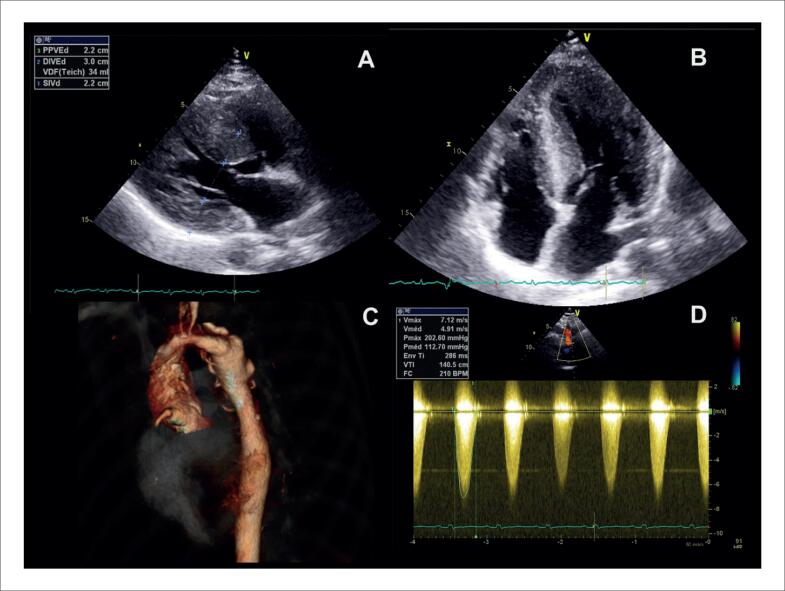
Imagens ecocardiográficas (A, B e D) e de angiotomografia de aorta torácica (C) de paciente com diagnóstico de arterite de Takayasu. A paciente apresenta hipertrofia miocárdica concêntrica de grau importante (A e B) secundária à estenose acentuada de arco aórtico, aorta torácica descendente, além de oclusão do tronco braquiocefálico e estenose acentuada da artéria carótida comum esquerda e artéria subclávia esquerda (C). Ao Doppler contínuo, foi medido gradiente máximo ao corte supraesternal em aorta descendente de 202 mm Hg.
